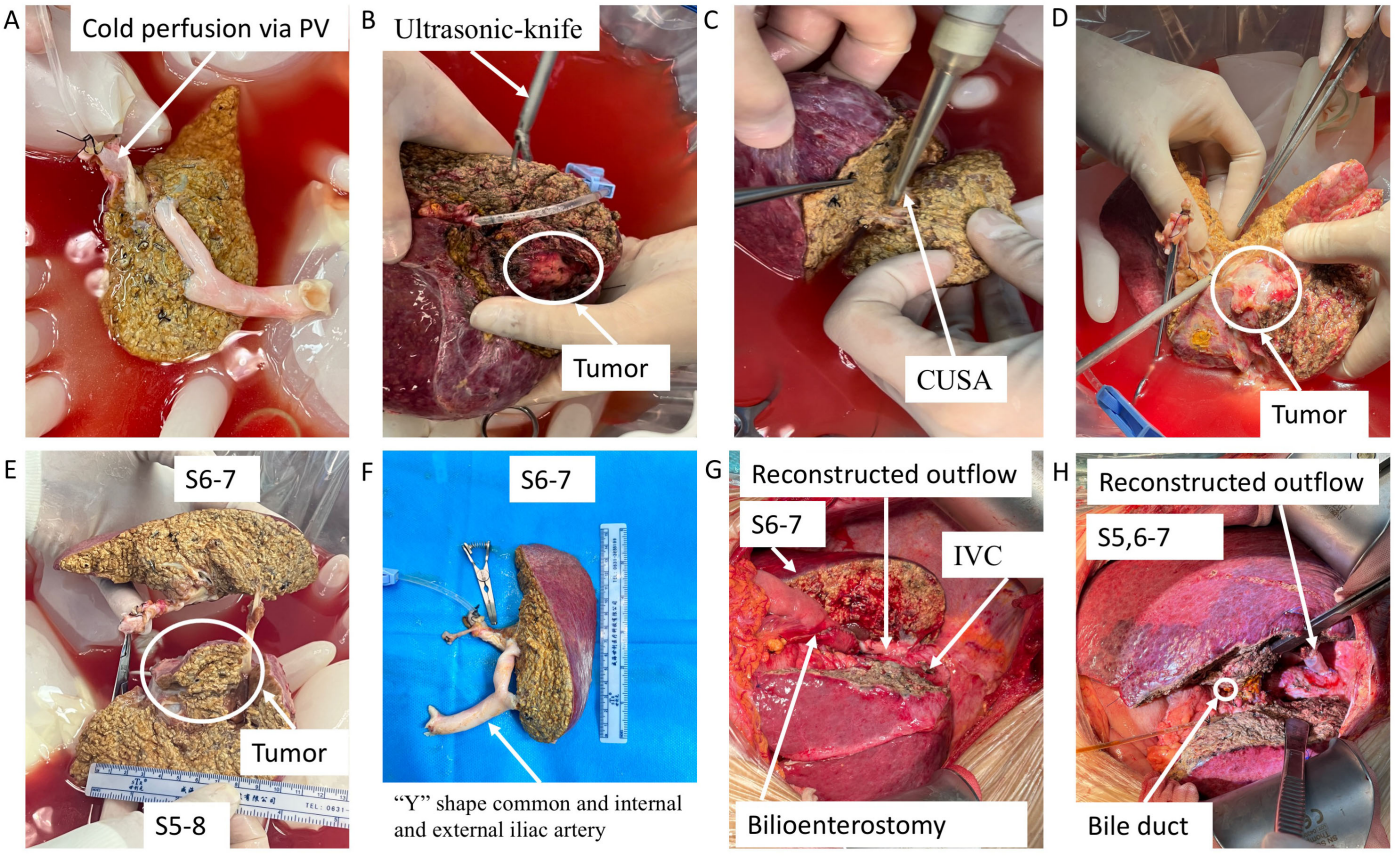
Key surgical steps of *ex vivo* tumor resection and partial liver autotransplantation and outflow reconstruction with pre-frozen allograft blood vessels. (A) The excised liver containing tumor was preserved in pre-cooling ice basin and flushed with HTK solution. (B–E) The tumor was completely removed *ex vivo* using CUSA, ultrasonic knife, and intraoperative ultrasonography. (F) The portal vein and outflow of the remaining liver segments were reconstructed using pre-frozen allograft vessels. (G, H) The tumor-free liver was autotransplanted into the anatomical position. PV, portal vein; HTK, histidine–tryptophan–ketoglutarate; CUSA, cavitron ultrasonic surgical aspirator.
